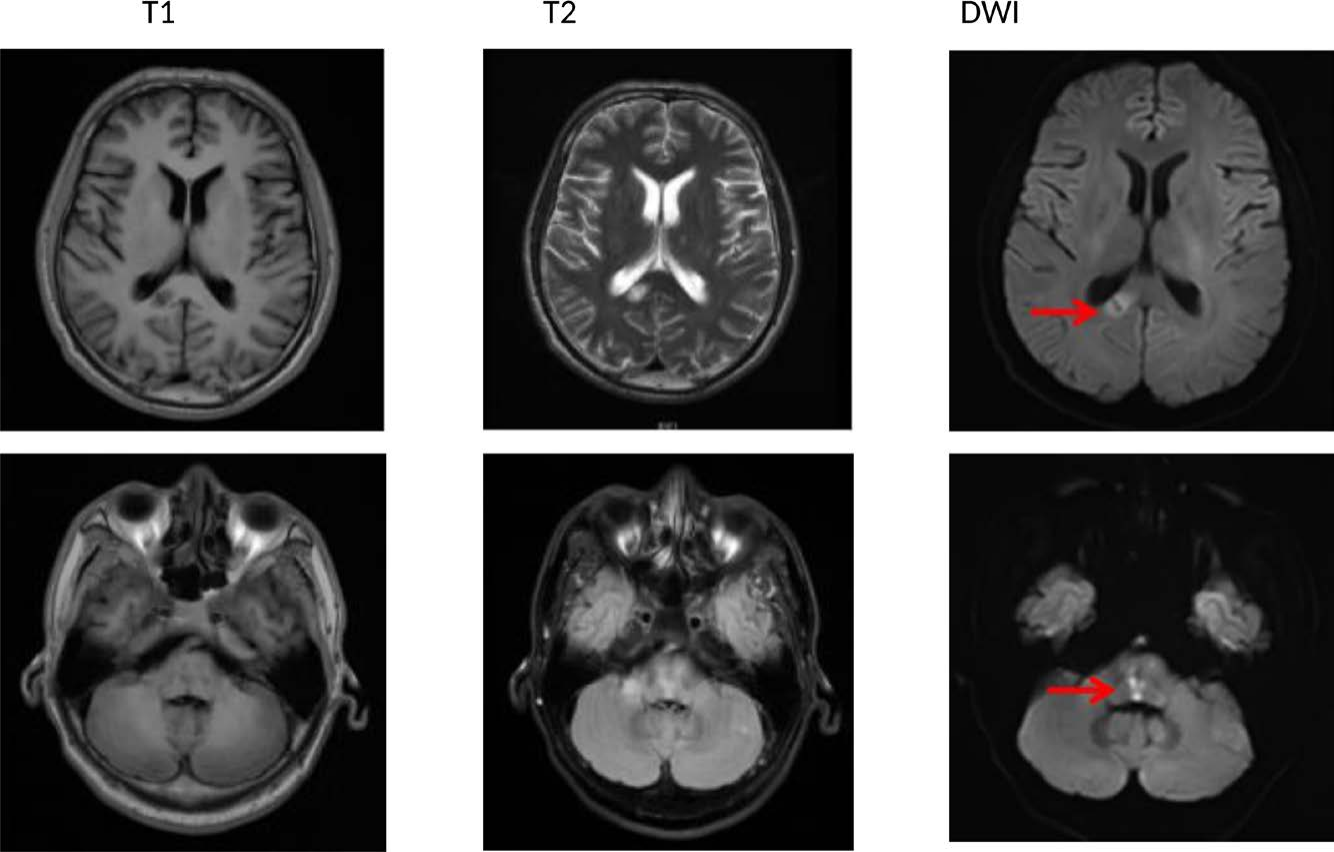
2021-11-11 The head MRI scan and diffusion examination revealed an acute and subacute lacunar infarction of the corpus callosum and pons and multiple lacunar cerebral infarctions in the brain.
